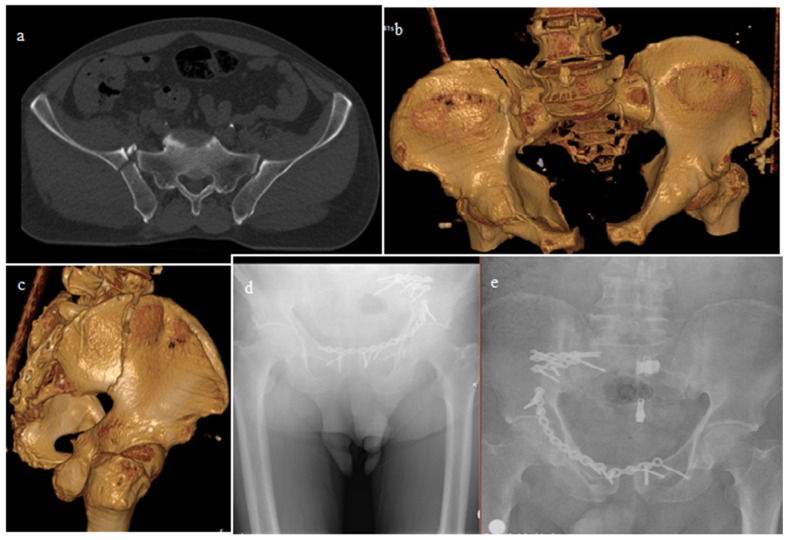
A 61-year-old male patient, experiencing a non-vehicle traffic accident, with a complex pelvic injury. Tomography images of crescent fracture dislocation and anterior pelvic injury (a–c). Fourth year control pelvis radiographs, anterior approach to the sacroiliac joint with two plate–screws (d,e).
